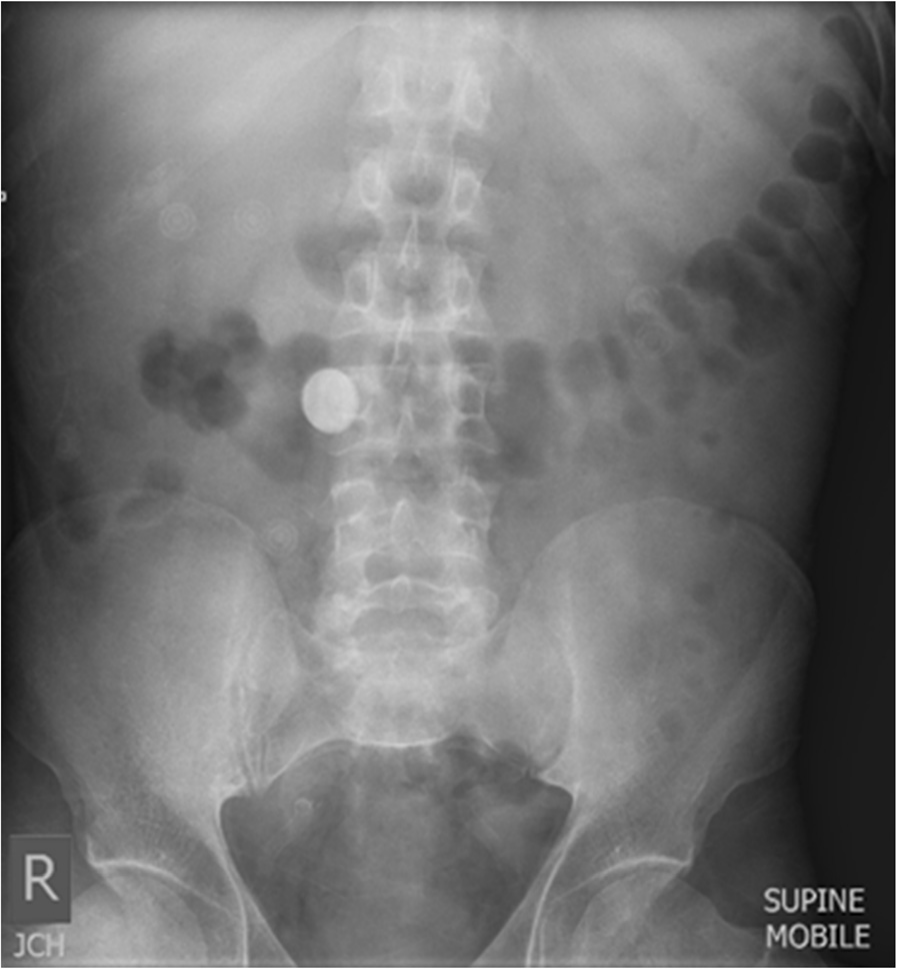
Plain abdominal radiographs showing the radiopaque coin at presentation.
